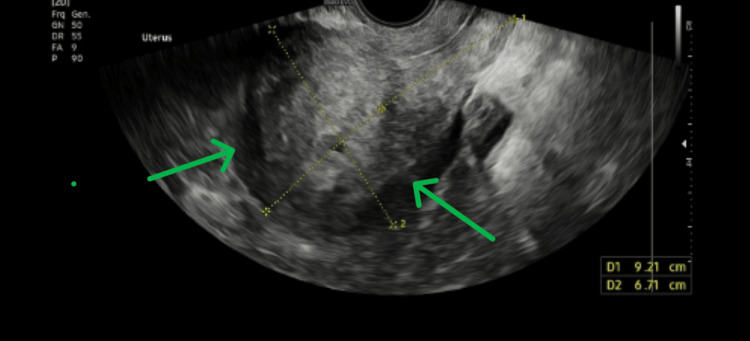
TVUS showing no obvious pelvic collections TVUS: transvaginal ultrasound scan
